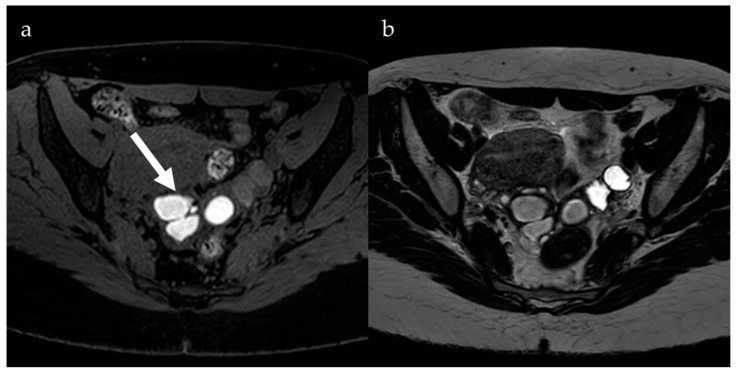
Right and left ovarian cysts showing high signal content on an axial fat-saturated T1-weighted image (a, arrow) and low signal on an axial T2-weighted image (b), suggesting hemorrhagic content. Both ovaries appear enlarged and in close relation, located in the rectouterine space (“kissing ovaries”).
